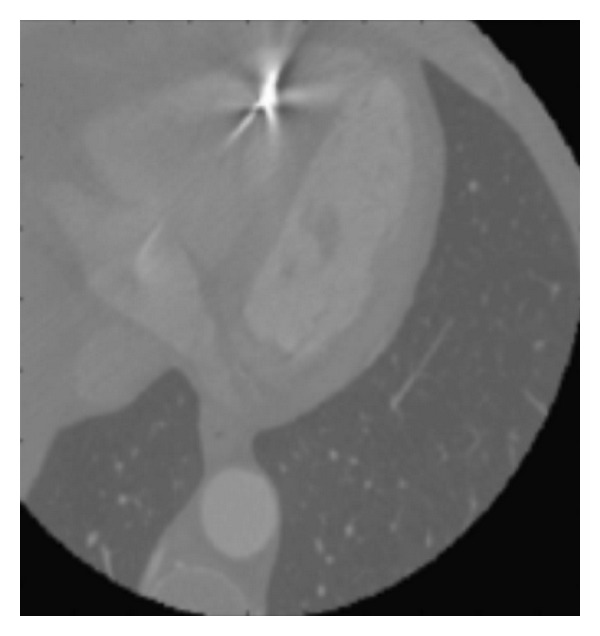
A transaxial image of the CT data volume in which an artifact caused by a metallic object is present.
